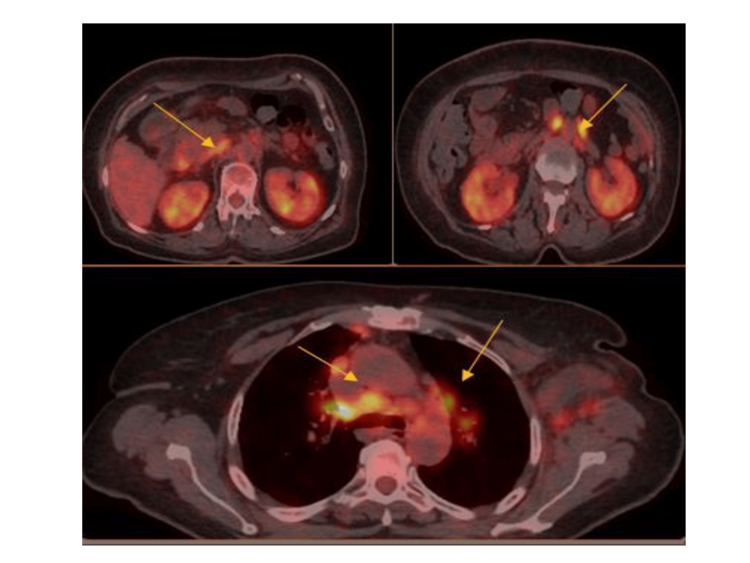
18-FDG PET/CT scan imaging 18-FDG PET/CT scan imaging revealed increased tracer uptake in the patient’s mediastinum, axillary, and abdominal lymph nodes. 18 FDG PET/CT: 18 F-fluorodeoxyglucose positron emission tomography
